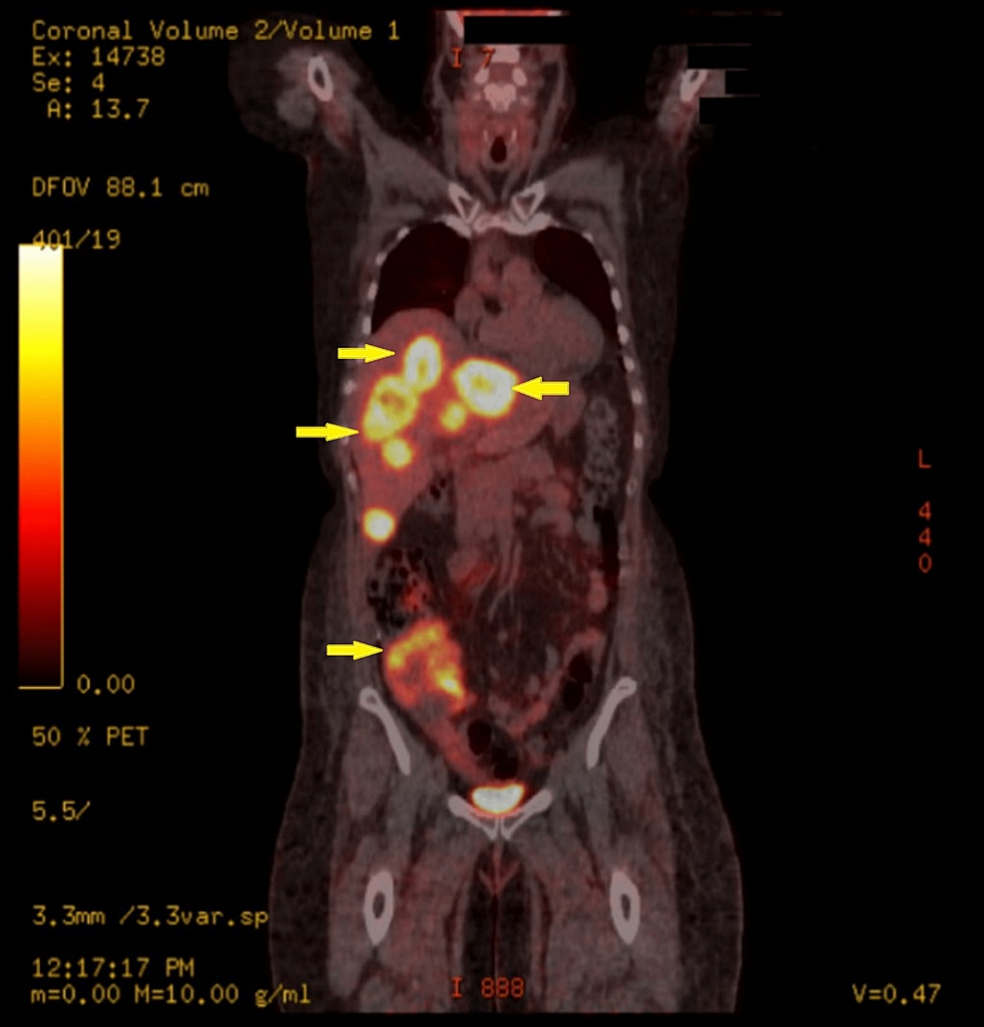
PET/CT demonstrated the presence of hypermetabolic lesions in the liver and right lower quadrant of the abdomen.
